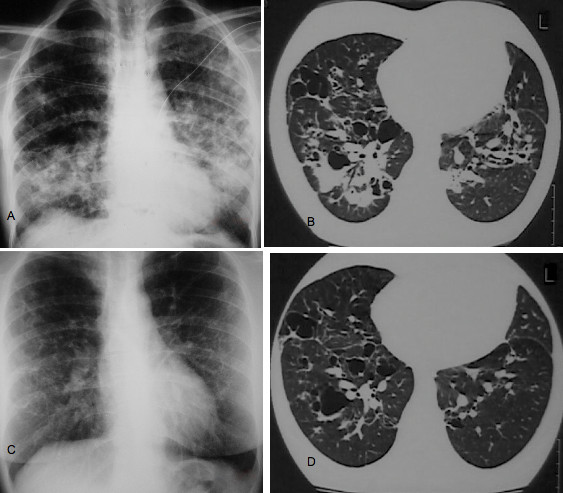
(A) Radiography of the chest at the time of bilateral pneumothorax revealing pulmonary Langerhans cell histiocytosis. (B) High-resolution computed tomography (HRCT) of the chest showing multiple cysts associated with nodules and extensive consolidation. (C) Radiography of the chest and (D) HRCT one year later showing significant improvement of the initial findings and regression of consolidation.
